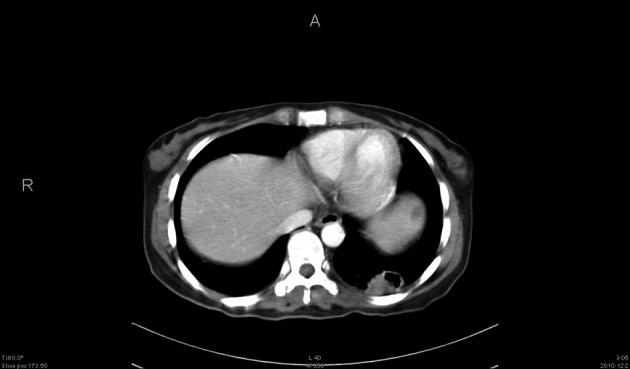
Initial transaxial computed tomographic scan showing a mass in the left lung.
